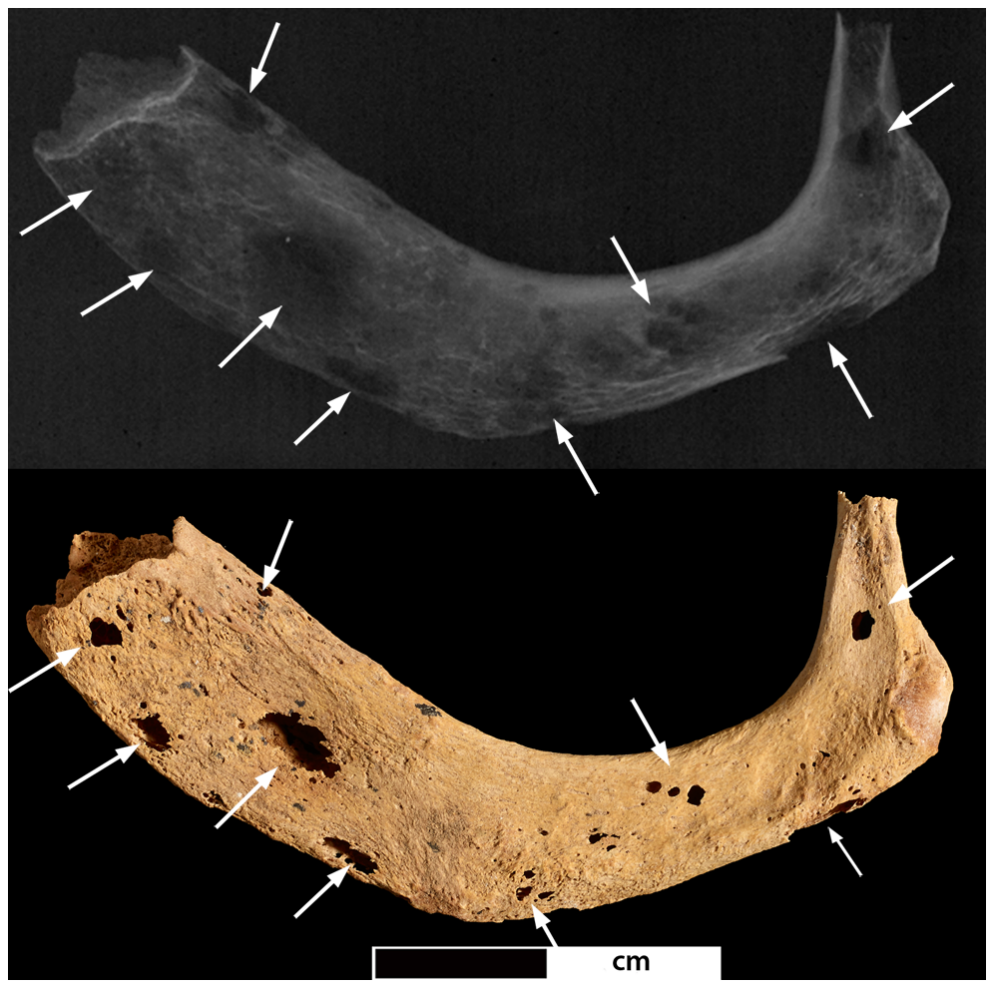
Lytic lesions in the first rib. Photo- and radiograph of the left first rib (superior surface. Arrows indicate location of the lesions.
